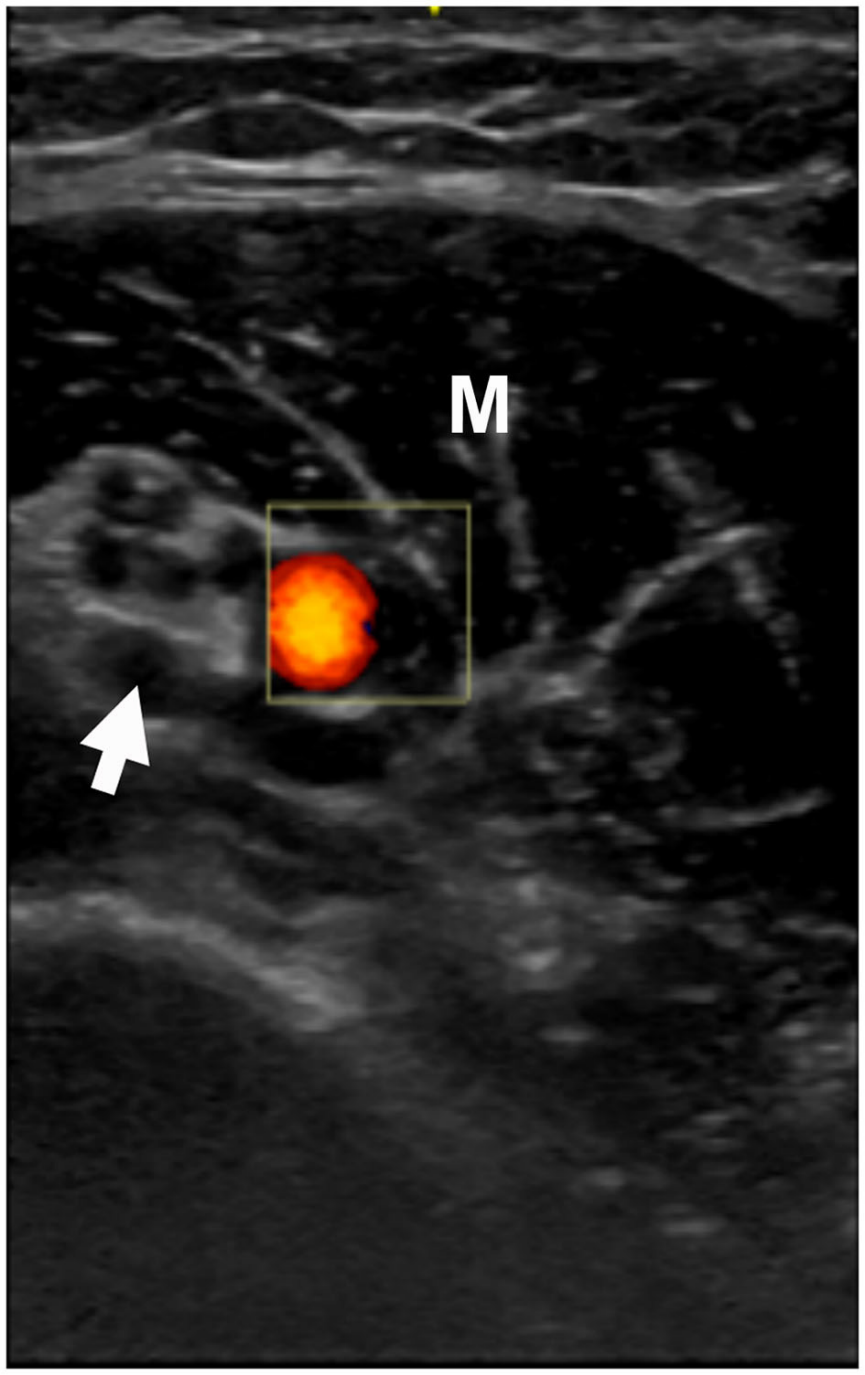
Patient #21: Ultrasound: short axis view at distal upper arm. Arrow points to the median nerve with hypoechoic enlarged fascicles and perineural thickening. The brachial artery is in orange color. M: Biceps muscle.
